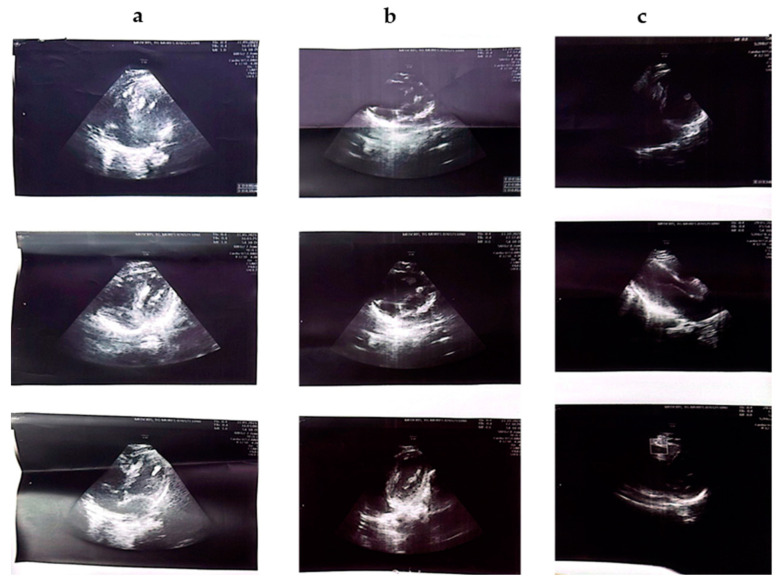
Cardiac ultrasound (no#3): ((a), left) 2021: multiple rhabdomyomas at the level of the left ventricular septum; ((b), middle) 2021: slightly reduced in volume; ((c), right) 2024: in regression.
